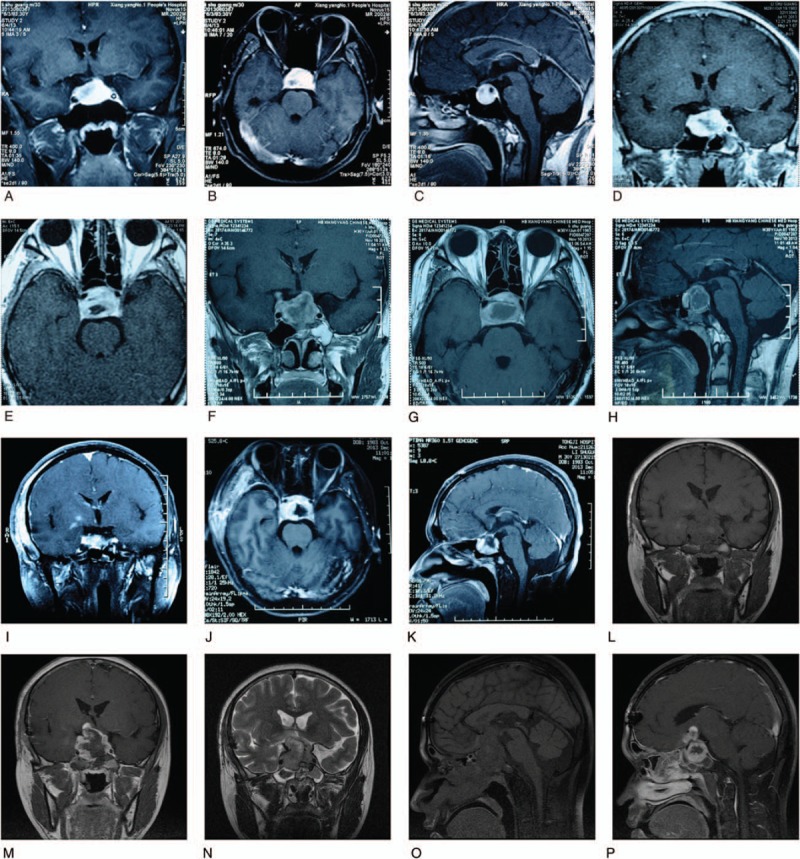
MRI for the abnormalities in the sellar region at different time points. A–C, Before the first surgery. A, Coronal-enhanced T1WI. B, Axial-enhanced T1WI. C, Sagittal-enhanced T1WI. D–E, Before γ-knife treatment. D, Coronal-enhanced T1WI. E, Axial T1WI. F–H, Before the secondary surgery. F, Coronal-enhanced T1WI. G, Axial T1WI. H, Sagittal-enhanced T1WI. I–K, After the secondary surgery. I, Coronal-enhanced T1WI. J, Axial-enhanced T1WI. K, Sagittal-enhanced T1WI. L–P, Before the third surgery. L, Coronal T1WI. M, Coronal-enhanced T1WI. N, Coronal T2WI. O, Sagittal T1WI. P, Sagittal-enhanced T1WI. MRI = magnetic resonance imaging.
